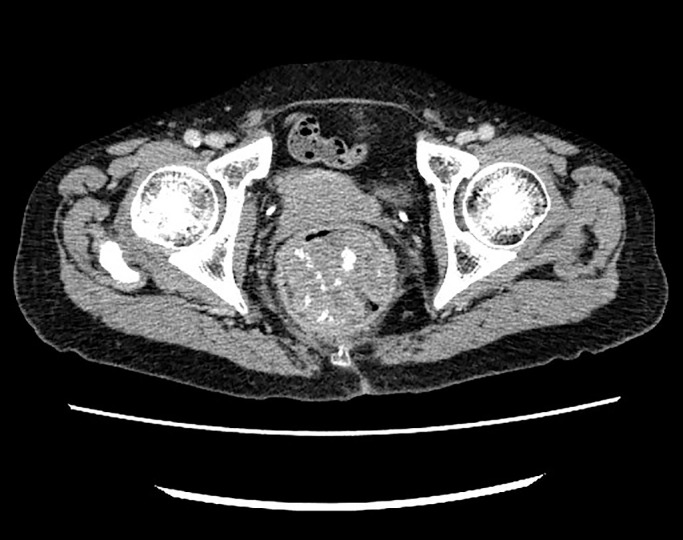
Abdominopelvic contrast-enhanced computed tomography shows irregular wall thickening on the pelvis with coarse heterotopic ossification.
